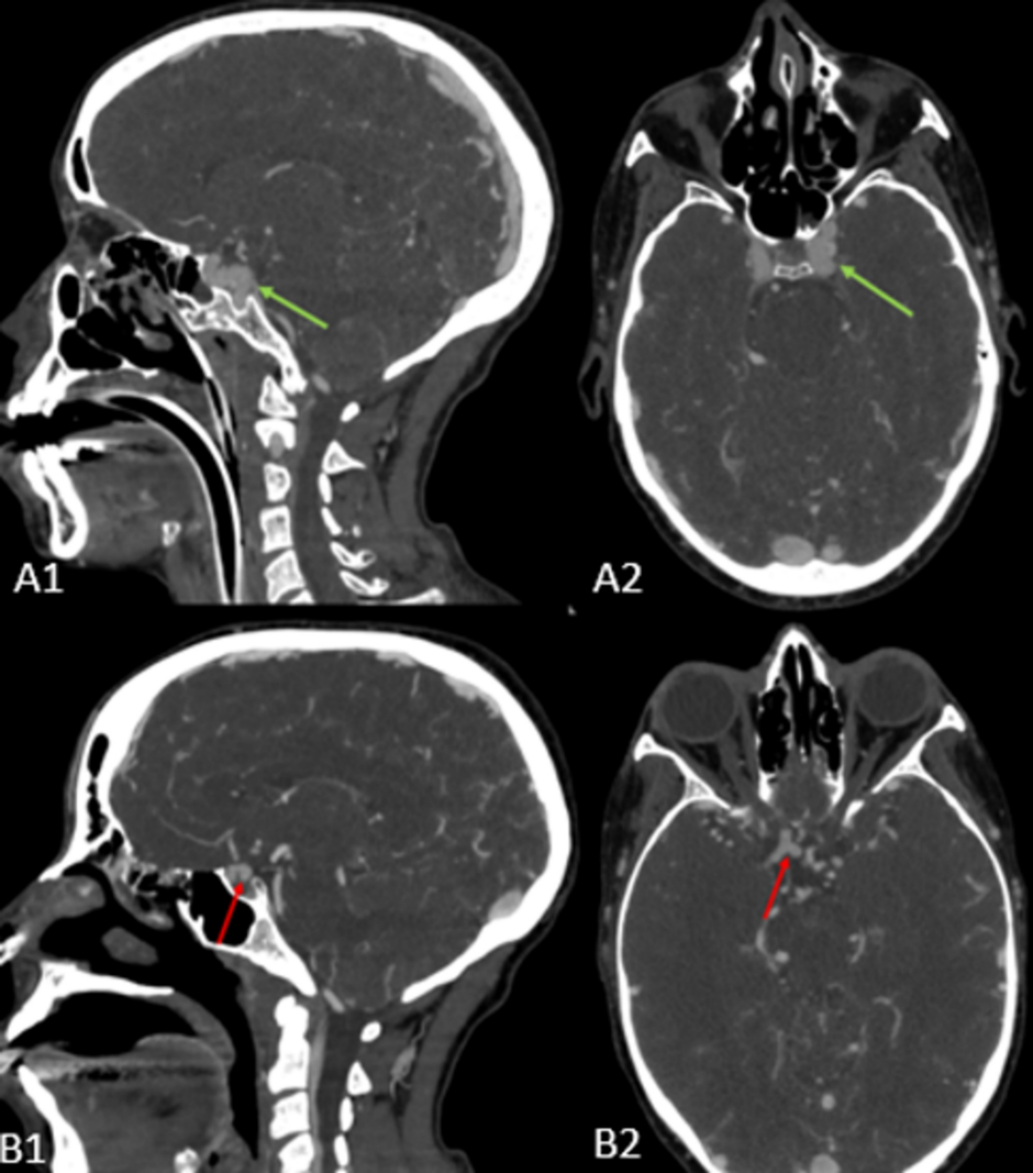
CTA in the coronal (A1, B1) and axial (A2, B2) planes Figure A shows fusiform aneurysm of the cavernous segment of the left internal carotid artery (green arrow). Figure B shows saccular aneurysm of the supraclinoid segment of the right internal carotid artery (red arrow) CTA: computed tomography angiography
